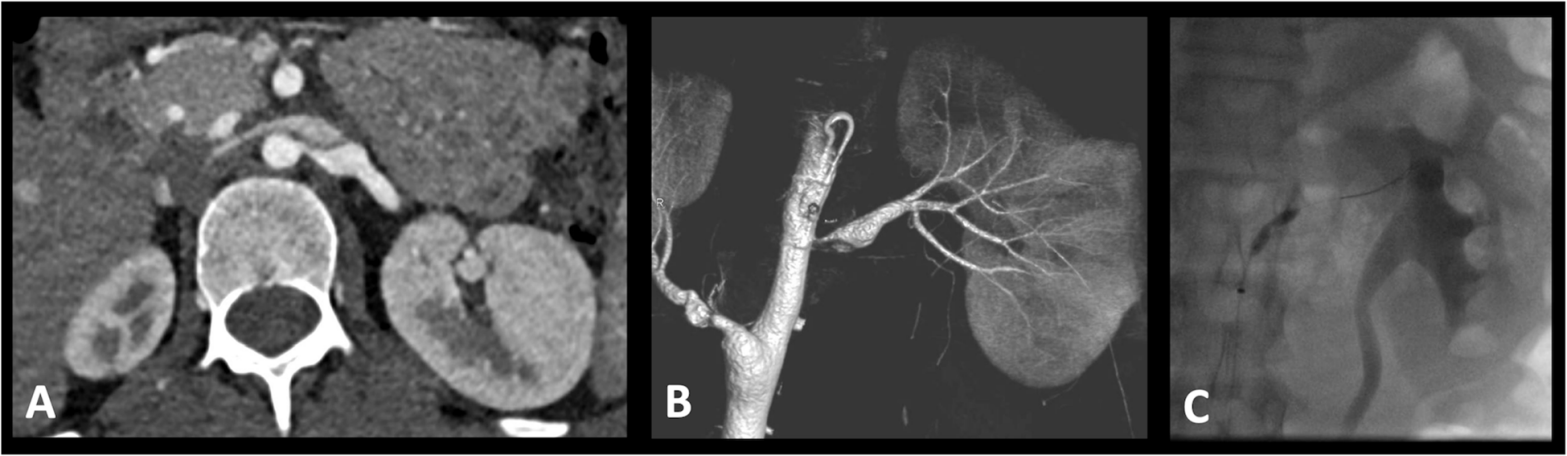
Renal Artery Stenosis Angioplasty. a Axial contrast enhanced CT angiogram image demonstrating a left renal artery stenosis with post stenotic dilation. b This was confirmed on subsequent rotational angiography and (c) at angioplasty by demonstration of a waist on the angioplasty balloon
